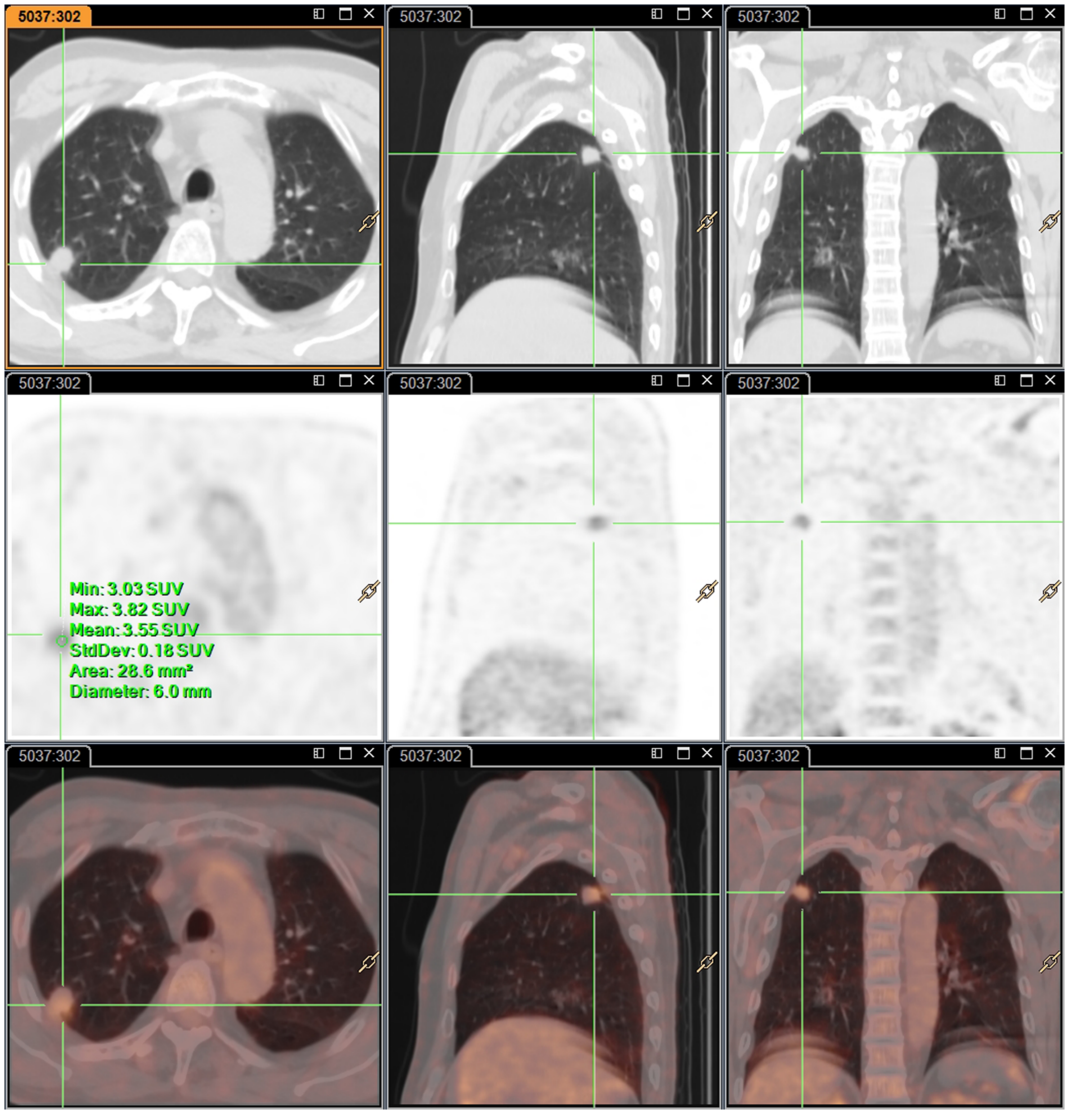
Representative images of PET-CT examination one-month post-surgery. there was no significant increase in metabolism in solid nodules in the upper lobe of the right lung.
